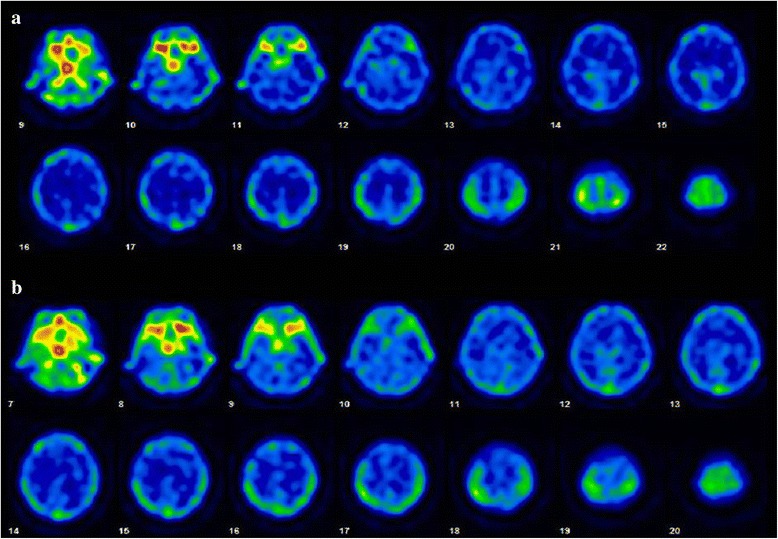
Thallium-201 single photon emission computed tomography at 11 days after admission. Early (a) and delayed (b) scans demonstrated no uptake in the lesions
